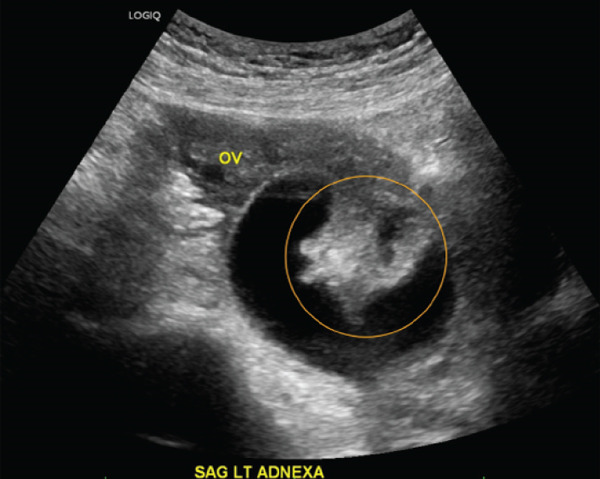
Pelvic ultrasound performed in the emergency department on HOD 1 demonstrates a “spoke and wheel” appearance, which is often associated with an abscess.
